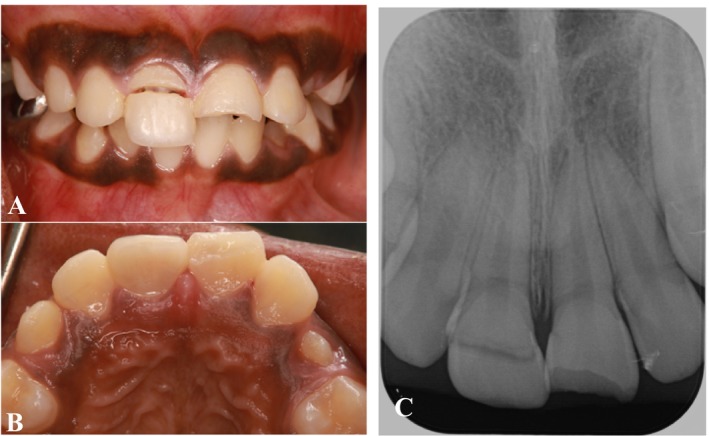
Preoperative clinical photos and radiograph. (A) Frontal view. (B) Maxillary occlusal view. (C) Preoperative periapical radiograph.
